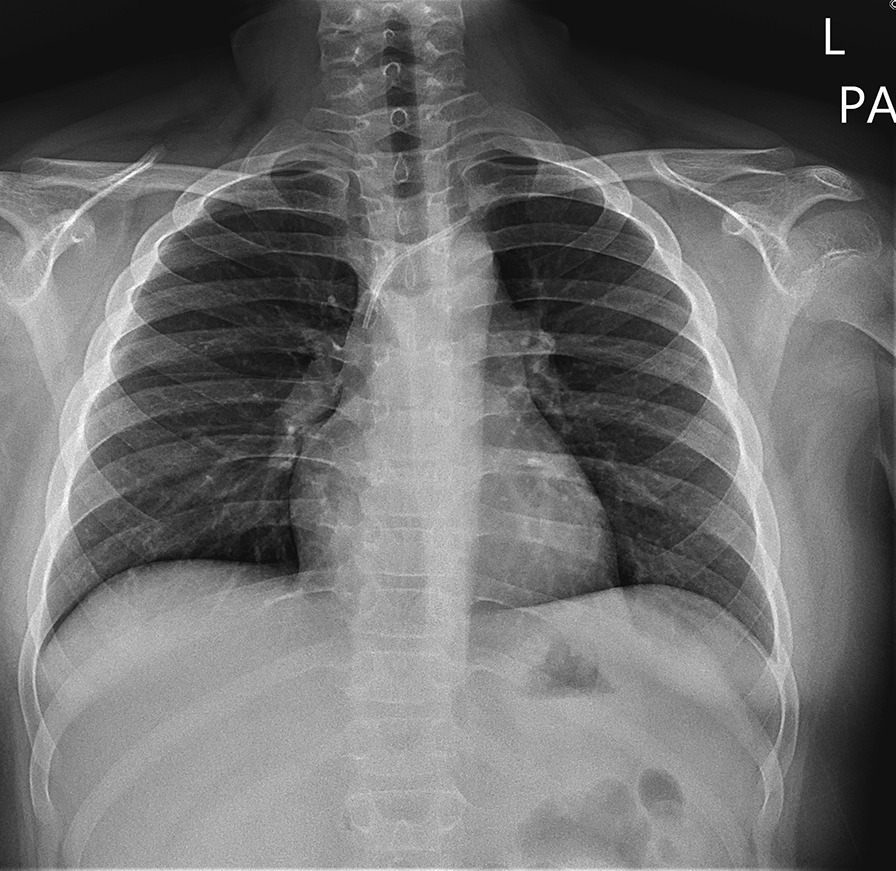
Postoperative chest X-ray showing a patient with a stuck fragment of a totally implantable central venous access port after removal operation
